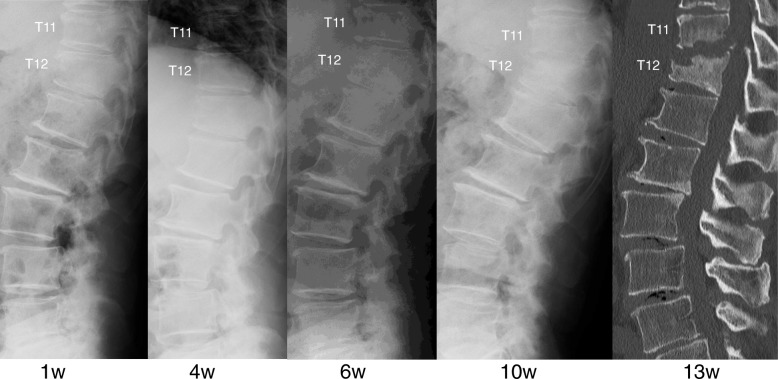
X-ray image showing narrowing of T11–T12 intervertebral disc space at 6 weeks and 10 weeks. A computed tomographic scan at 13 weeks showed numerous irregular osteolytic cavities in T11 and T12 vertebral bodies and destruction of the inferior endplate of T11 and the superior endplate of T12
